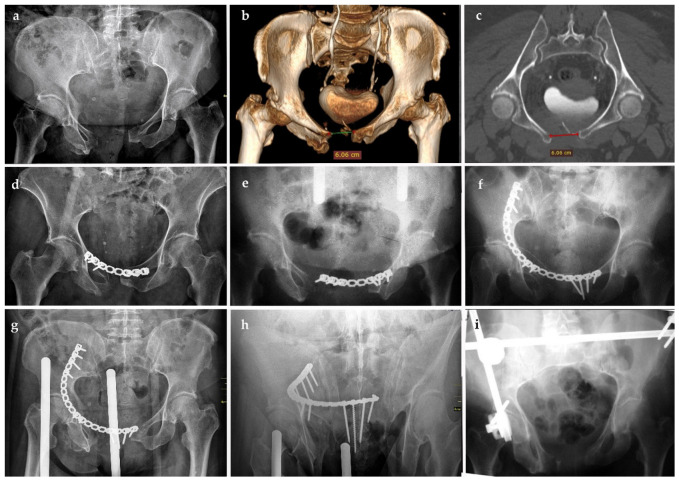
Example of acute symptomatic mechanical failure requiring revision surgery: preoperative pelvic radiograph (a), 3D CT reconstruction (b), and axial CT scan (c) showing severe pubic diastasis of 6.06 cm; immediate postoperative pelvic radiograph (d) showing adequate reduction in the pubic symphysis; radiograph obtained 4 days postoperatively (e) demonstrating pull-out of the plate and screws from the right pubic bone after sudden acute pain, consistent with early mechanical failure; post-revision radiograph (f) showing fixation with a longer reconstruction plate extending to the iliac wing; follow-up radiographs (g,h) showing contralateral plate and screw pull-out after a second acute painful episode; final radiograph after second revision (i) showing implant removal and stabilization with an external fixator.
